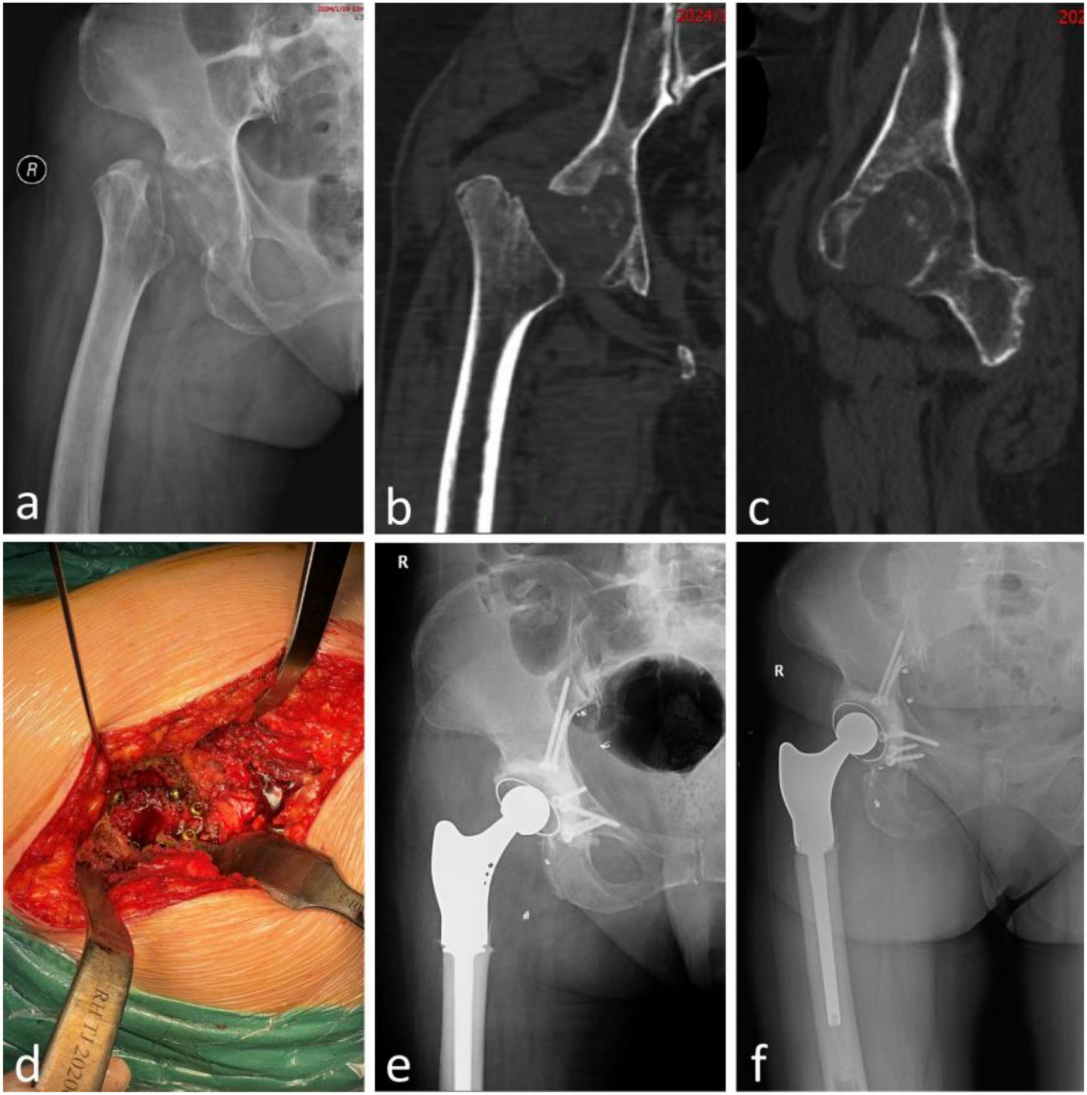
Patient 2 with Gorham–Stout disease underwent acetabular reconstruction using the screw-and-cement pile technique with a standard polyethylene cemented cup, together with total hip arthroplasty and partial proximal femoral replacement. (a) Preoperative anteroposterior (AP) hip joint radiograph demonstrating extensive acetabular destruction with loss of normal contour and absence of the femoral head and neck; (b, c) preoperative computed tomography confirming the same defects; (d) intraoperative view showing multiple screws inserted from within the acetabulum and directed radially outward, intentionally left partially proud to function as “piles”; (e) postoperative AP hip joint radiograph; (f) AP hip joint radiograph at 16 months demonstrating maintained component position without screw back-out, cup migration, or loosening.
